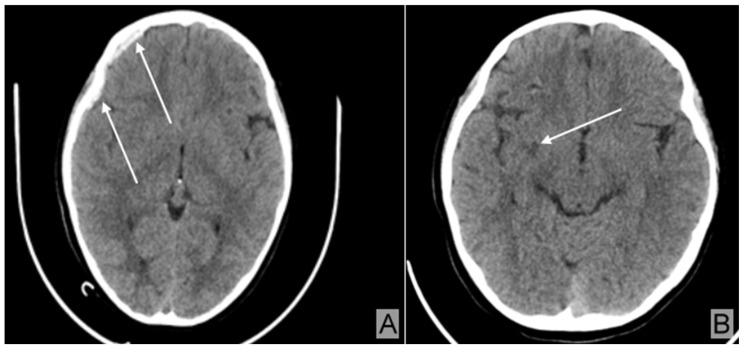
Skull CT: (A) A spontaneously hyperdense area measuring 60 mm in length and 4 mm in maximum thickness (arrows), exhibiting the characteristics of an acute subdural hematoma. (B) A spontaneously hypodense area measuring 4.8 mm in size (arrow) in the white matter of the right frontal region, suggestive of diffuse axonal injury.
